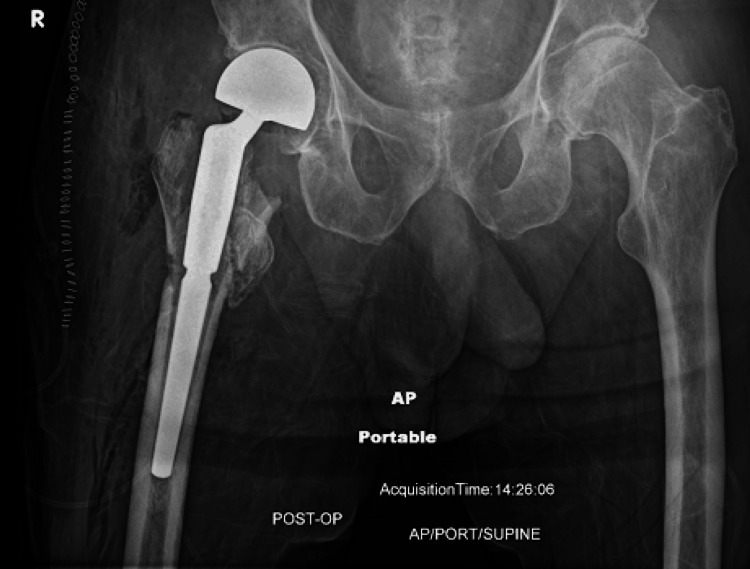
Postoperative anteroposterior radiograph of the right hip Anteroposterior radiograph of the right hip following hemiarthroplasty demonstrating appropriate positioning of the femoral prosthesis without complication.
